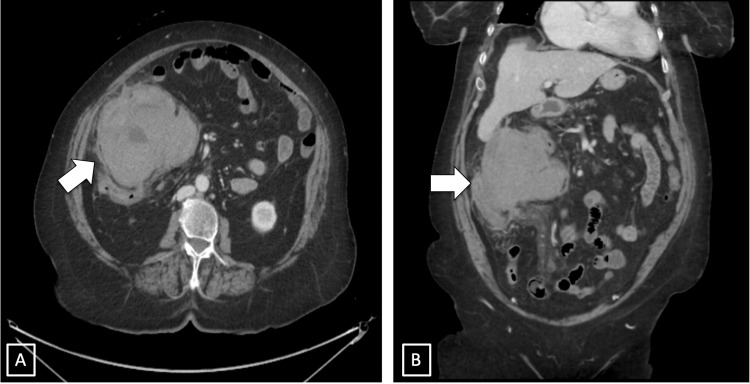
CT abdomen (A) axial view showing a heterogenous mass (large arrow) inseparable from the right colon. (B) Coronal view heterogenous mass (large arrow) away from liver bed.
